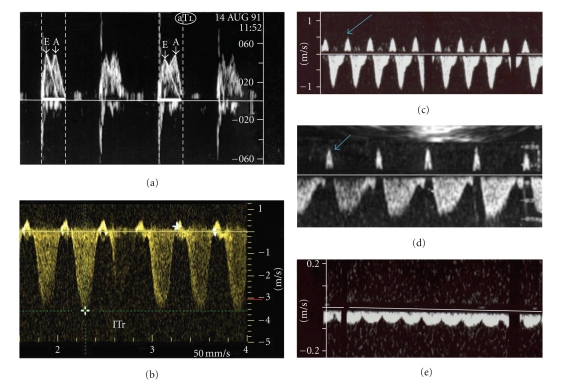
Doppler findings in fetuses with DCM and fetal hydrops: (a) The tracing of the tricuspid valve (T) shows a reduced systolic A-wave, with respect to the diastolic E-wave; (b) holosystolic tricuspid regurgitation (ITr); (c) a reverse systolic flow of the inferior vena cava (small arrow); (d) a reverse systolic flow of ductus venosus (small arrow); (e) the fluctuation of the umbilical vein.
